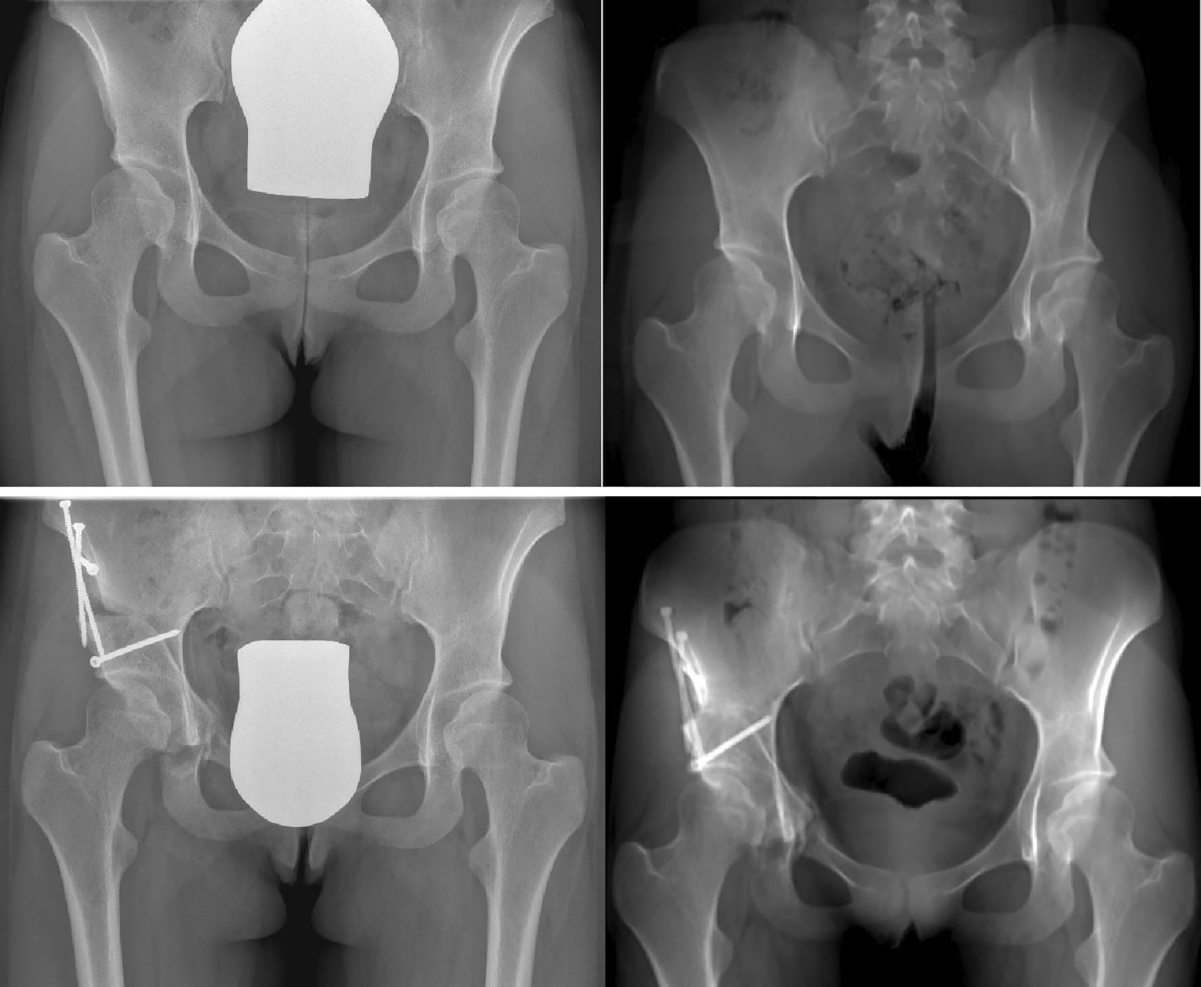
Side-by-side comparison of conventional radiographs on the left and radiograph-like reconstructions on the right depicting the excellent quality
